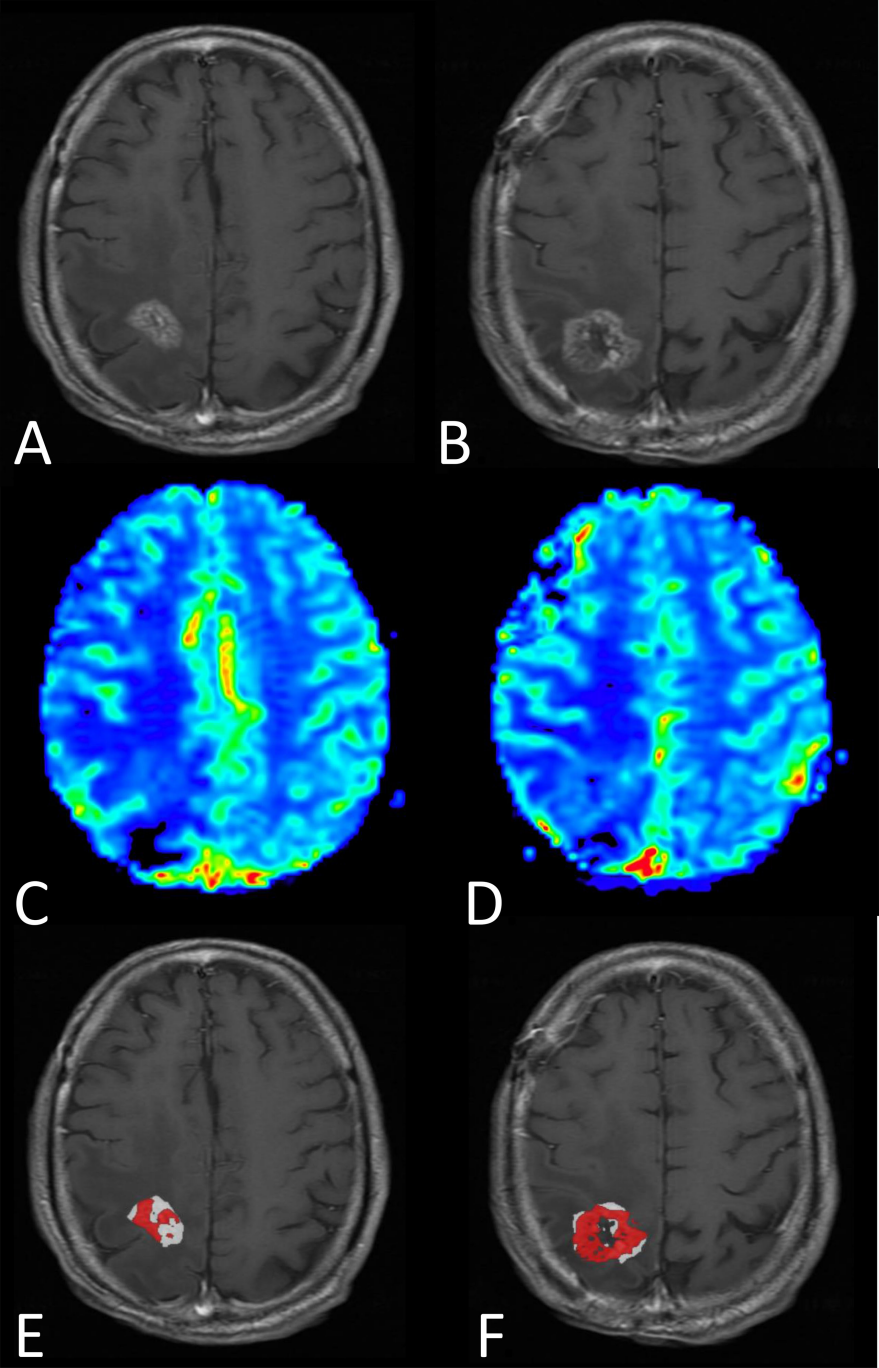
MRI results from a male patient diagnosed four months earlier with glioblastoma, IDH-wildtype, and treated with surgery followed by standard-of-care radiotherapy and concomittant temozolomide. Progression was questioned at the time of this MRI exam (three days prior to re-do surgery). (A, B) Post-contrast *T*_1_-weighted and (C, D) rCBV maps shown for two representative images slices. (E, F) FTB were created and show a mixture of tumor (red) and treatment effect (white), which is consistent with the pathologic diagnosis of tumor and treatment effect, respectively. Note that without the previously determined rCBV thresholds to distinguish tumor from treatment effect, the “blush” of higher rCBV noted on the rCBV maps would be difficult to interpret.
